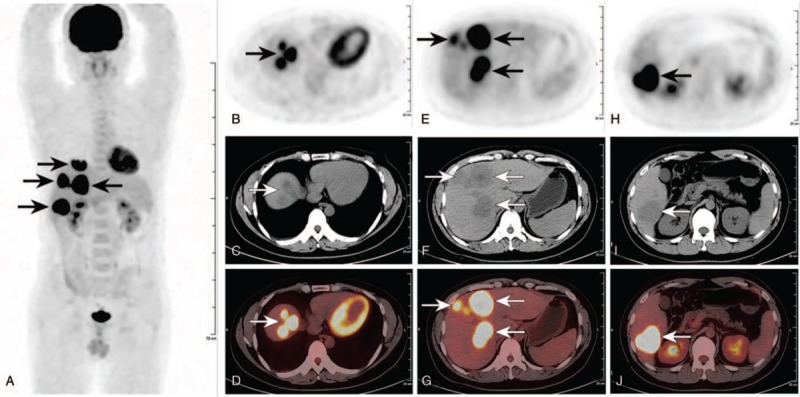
The preoperative ^18^F-FDG PET/CT images. (A) The MIP image showed multiple FDG-avid lesions (black arrows) confined to the liver without abnormal uptake in any other tissues or organs. (B–J) Figures displayed from upper to lower axial ^18^F-FDG PET, CT and PET/CT fusion, respectively. These images revealed multiple well-defined intense FDG-avid lesions mainly involved the right lobe and partial left medial segments of the liver (B–J, black and white arrows). Among these FDG-avid lesions, the maximum lesion located in segment VI with the diameter of 49 mm and SUVmax of 24.9 (H–J, black and white arrows). ^18^F-FDG = ^18^F-fluoro-2-deoxy-D-glucose, MIP = maximum intensity projection, PET/CT = positron emission tomography/computed tomography, SUVmax = maximum standardized uptake value.
